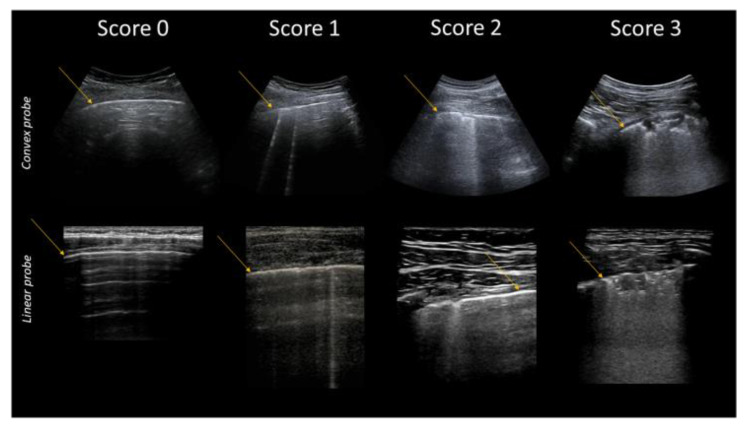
Classification of pathological lung ultrasound findings in COVID-19 patients. Arrows indicate the pleural line. Top: convex probe. Bottom: linear probe.
